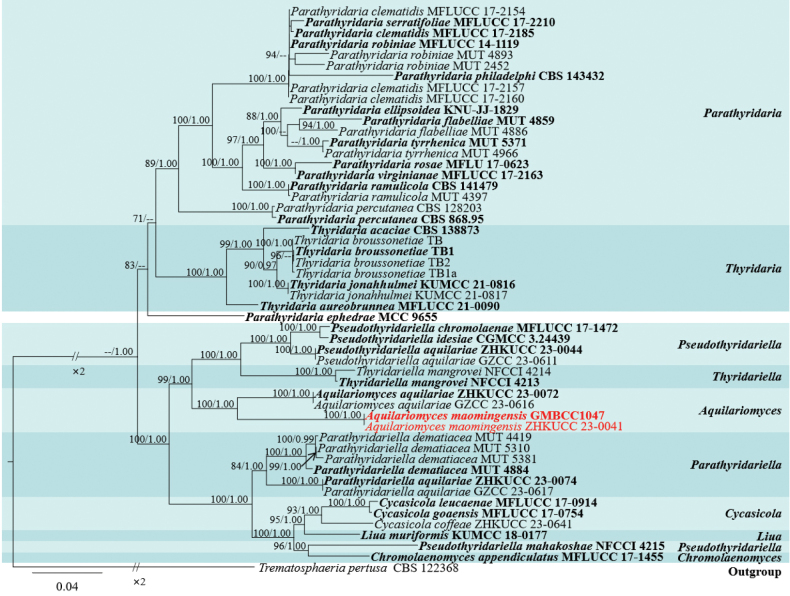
Phylogram generated from maximum likelihood (ML) analysis based on combined LSU, ITS, SSU, *tef*1-α, and *rpb*2 sequence data of 52 taxa, which comprised 4387 base pairs of LSU = 967, ITS = 516, SSU = 872, *rpb*2 = 1024, *tef*1-α = 1008. The best-scoring RAxML tree with a final likelihood value of -31596.745436 is presented. The matrix had 2090 distinct alignment patterns, with 47.13% of undetermined characters or gaps. Estimated base frequencies were as follows: A = 0.249245, C = 0.257277, G = 0.271627, T = 0.221851; substitution rates: AC = 1.210949, AG = 2.948777, AT = 1.318881, CG = 0.897105, CT = 6.444599, GT = 1.0; gamma distribution shape parameter *α* = 0.441696. Bootstrap support values for ML equal to or greater than 70% and clade credibility values equal to or greater than 0.90 from Bayesian inference analysis are labelled at each node. The tree is rooted with *Trematosphaeriapertusa* (CBS 122368). The new isolates are indicated in red, and the ex-type strains are in bold.
